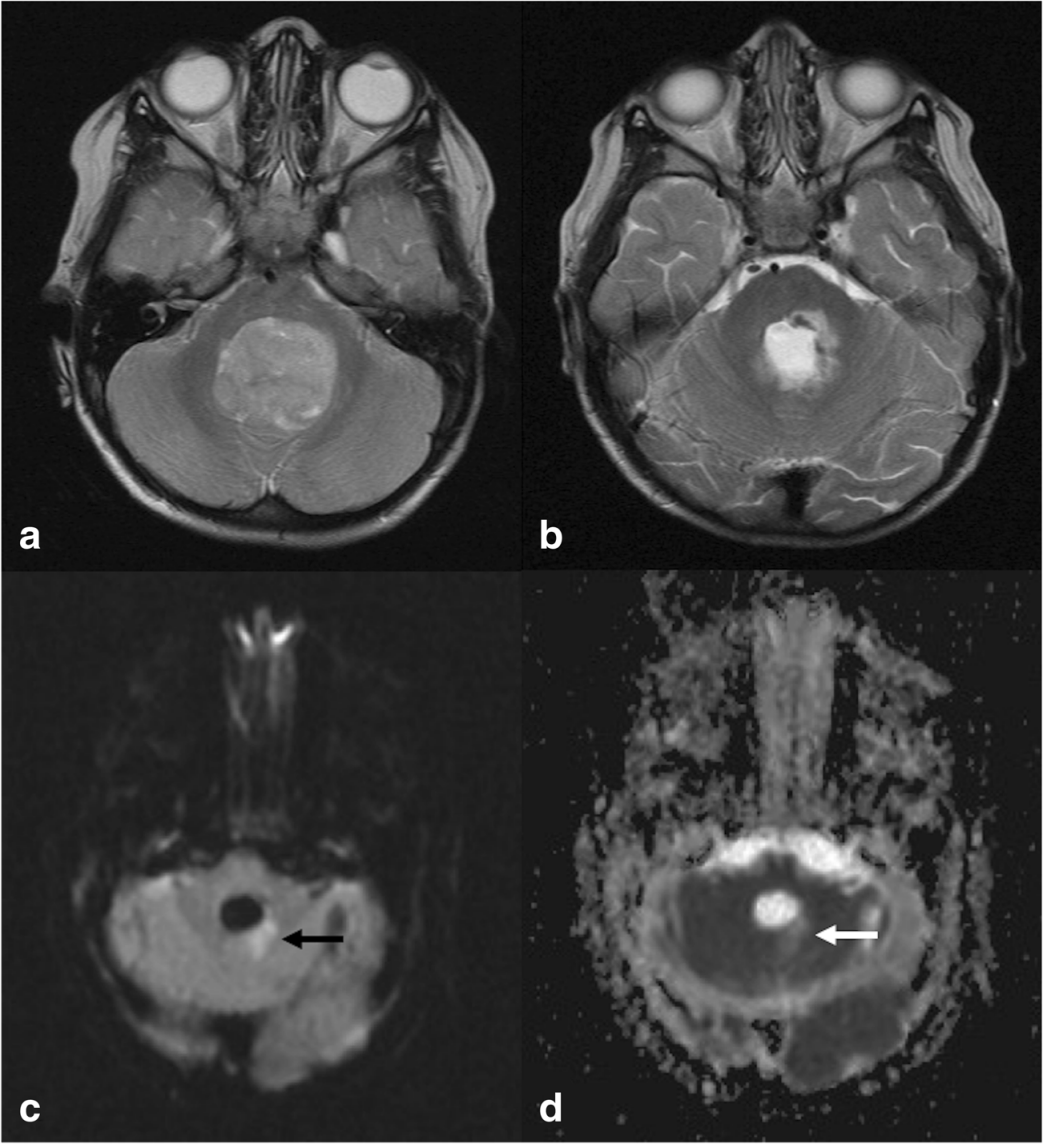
Images from a child who developed pCMS. a Pre-operative axial T2-weighted MRI showing tumour occupying the fourth ventricle, with distortion and signal change in the left dentate nucleus region. b Early post-operative axial T2-weighted MRI showing left-sided dentate nucleus signal change (which also extended to the left SCP on other slices). c Post-operative DWI (b = 1000) showing left-sided dentate nucleus signal change (black arrow), also evident in d ADC map at same position (white arrow)
